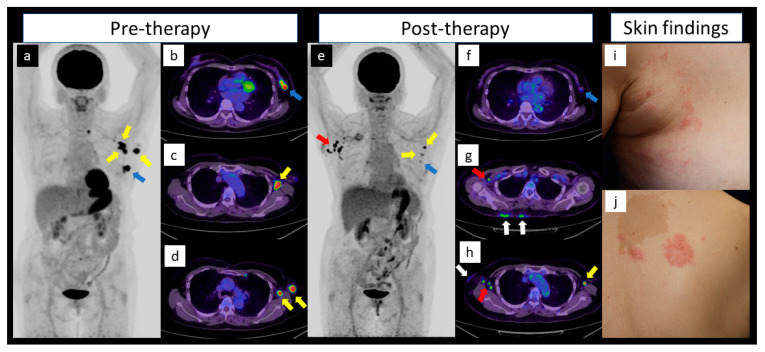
(a) Maximum-intensity projection (MIP) and (b–d) transaxial 18F-Fluorodeoxyglucose positron-emission tomography/computed tomography (18F-FDG PET/CT) images before therapy revealed (a,b: blue arrows) intense, hot uptake in the left breast mass indicating cancer, and (a,c,d: yellow arrows) left axillary lymph nodes indicating metastasis. (a) No other areas of uptake indicated distant metastasis. (e) MIP and (f–h) transaxial images of 18F-FDG PET/CT after therapy showed shrinking and decreased uptake in the (e,f: blue arrows) cancerous mass and (e,h: yellow arrows) left axillary lymph node metastasis, whereas (e,g,h: red arrows) enlarged lymph nodes with high FDG uptake appeared in the right axilla. (g,h: white arrows) Thickening of the skin on the right upper hemithorax with mild FDG uptake was also observed. The patient had painful vesicular eruption on the (i) front to (j) back of the right upper hemithorax, which was diagnosed as active herpes zoster.
